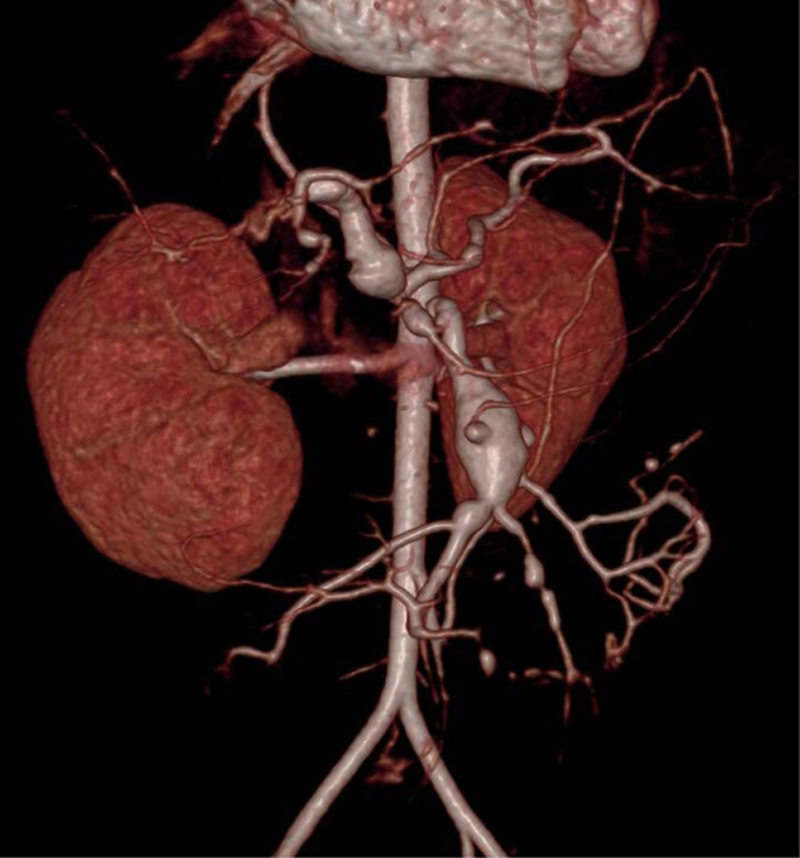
Three-dimensional computed tomography angiography, with fusiform aneurysms of superior mesenteric artery and hepatic artery.
